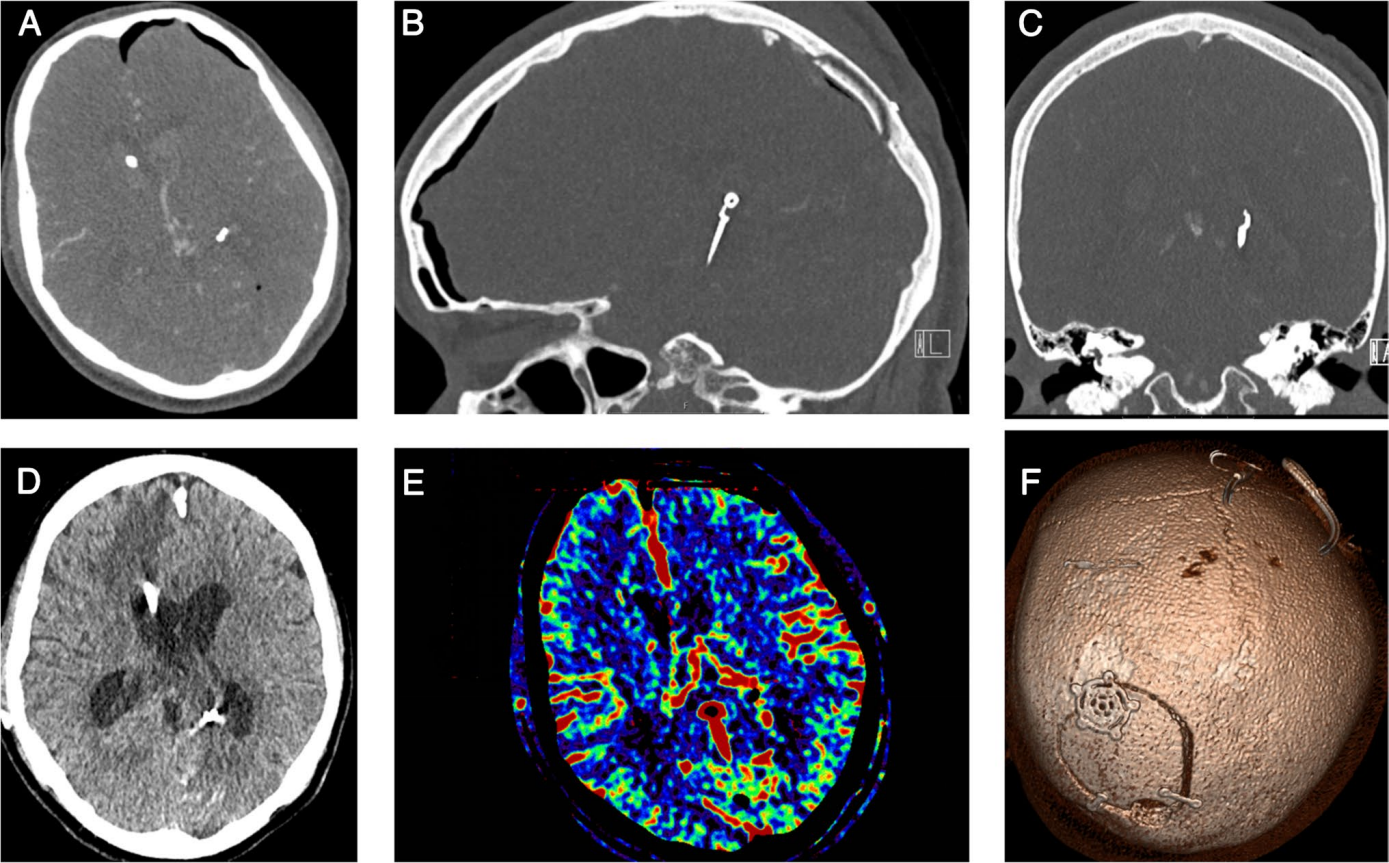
Postoperative images of case 2. A–C Axial, coronal, and sagittal CT images immediately after surgery demonstrating the clips’ position. D CT scans 5 days after surgery show the hematoma in the ventricles had disappeared. Red arrows show the EVD tube. E Postoperative CT perfusion scan shows no acute perfusion deficits. F 3D reconstructive image showing the craniotomy
